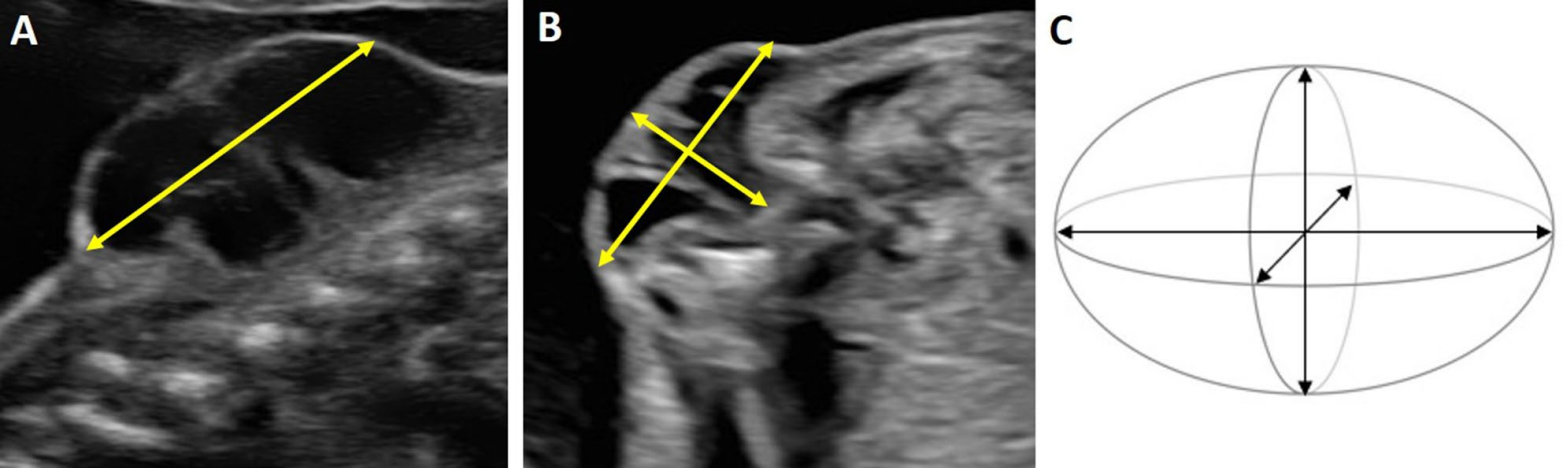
Volume of the myelomeningocele: ultrasound measurements. (A) Sagittal view of a myelomeningocele at 20 weeks of gestation: length. (B) Transversal view of a myelomeningocele at 20 weeks of gestation: width and depth. (C) Volume of the myelomeningocele: prolate ellipsoid volume: length × width × depth × 0.52.
